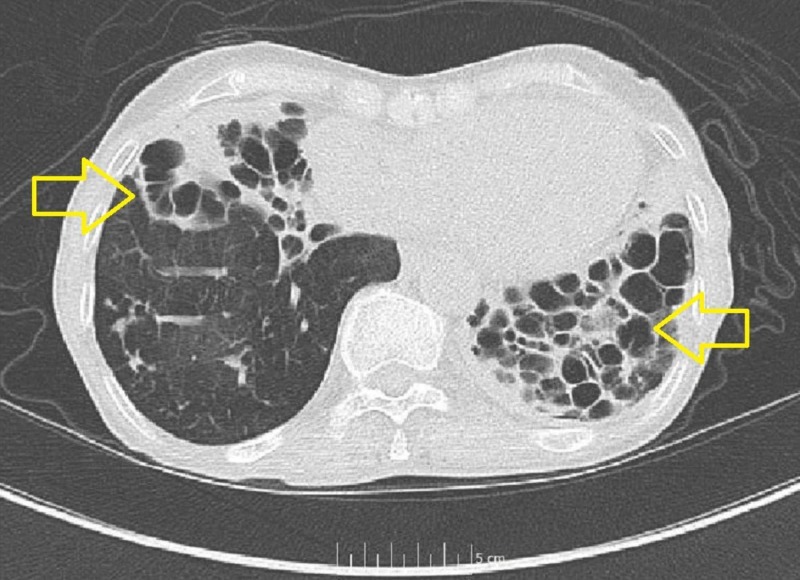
Repeat CT chest without contrast, read as extensive long-standing cystic bronchiectasis and fibrosis with overall appearance stable and unchanged from previous imaging.
